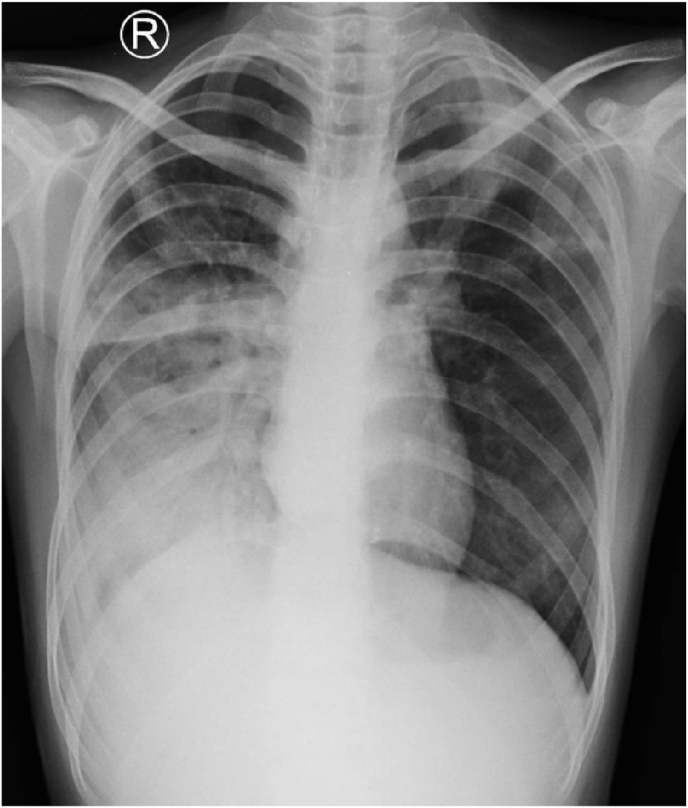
Chest X-Ray of a 14-years-olf girl with Pre-XDR TB. It shows opacity at the right hilar, lobulated infiltrate in the left apex, nodular at left hilar, and lymph node enlargement at bilateral peri-hilar.
